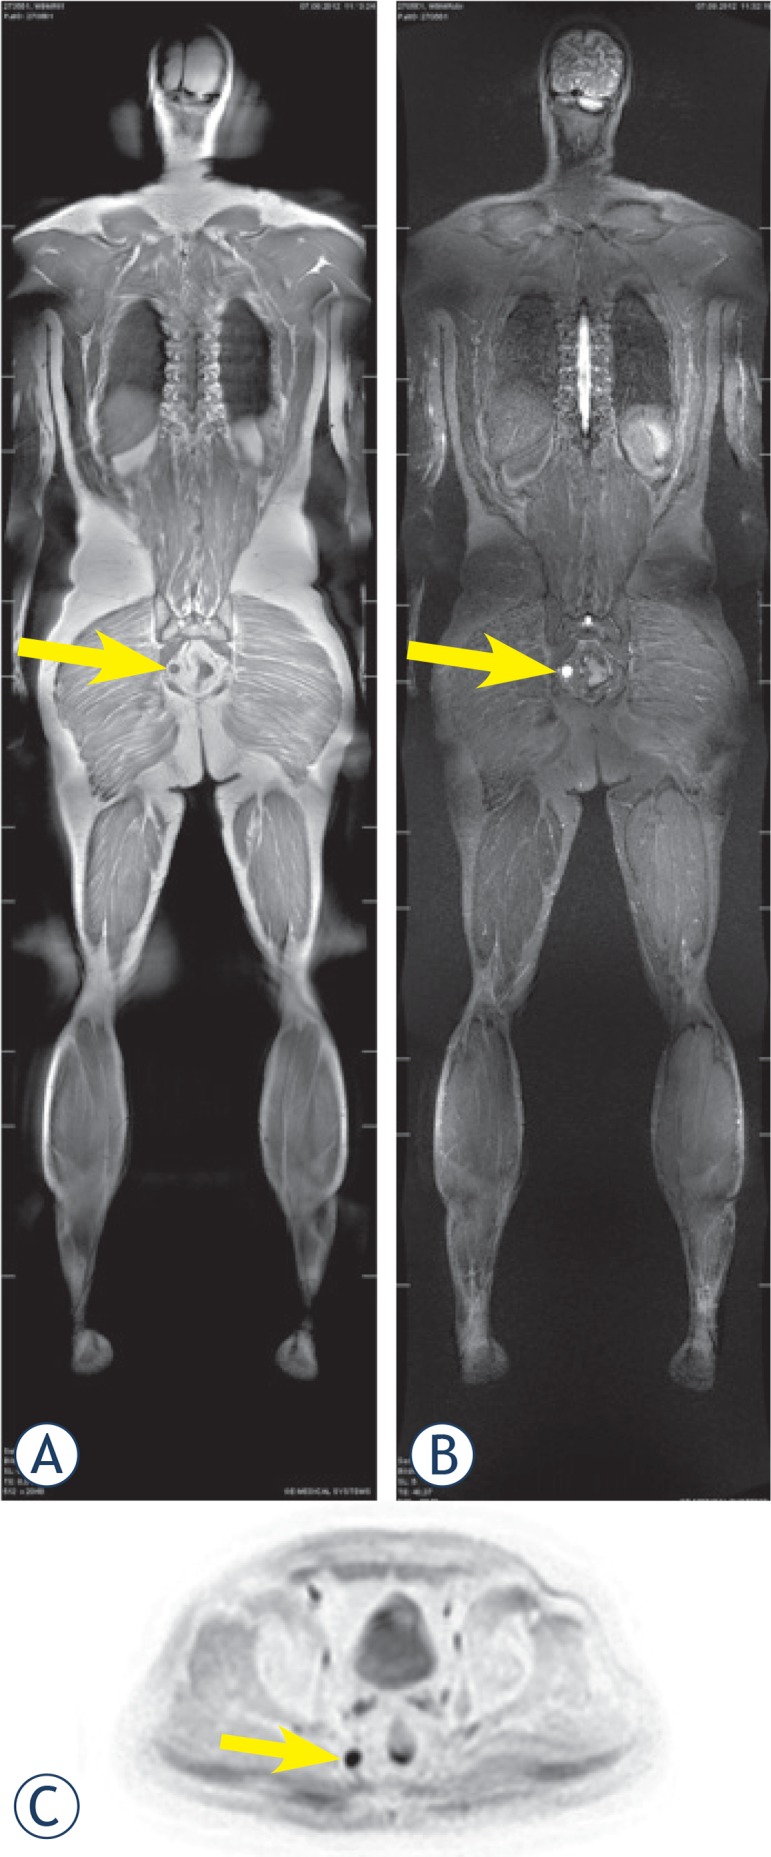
Whole-body MR image of a 52-year-old male patient with history of sleep disorder, night sweats, hypercholesterinaemia and previous smoking. Note the right pararectal 16×14 mm mass (arrows) on the coronal (A) T1 (hypointense signal) and (B) STIR images (hyperintense signal); (C) diffusion weighted (DWI) axial image indicating a restricted diffusion. Inverted DWI image is similar to a PET image.
